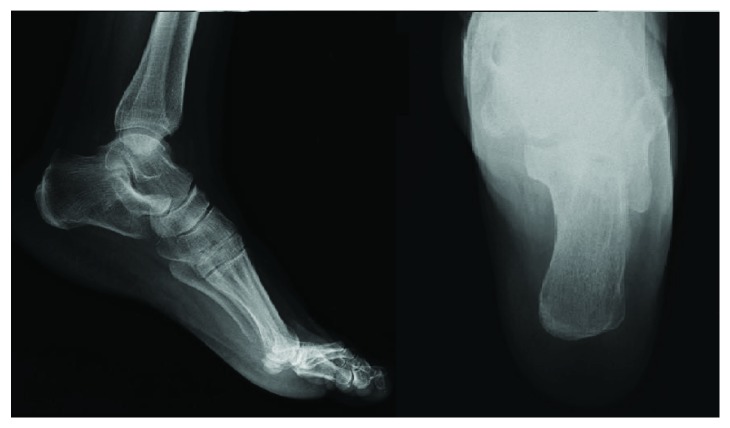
Retrospective assessment of the preoperative plain radiographs of the left foot shows a 3 cm oval lytic lesion in the anterior aspect of the calcaneum.
